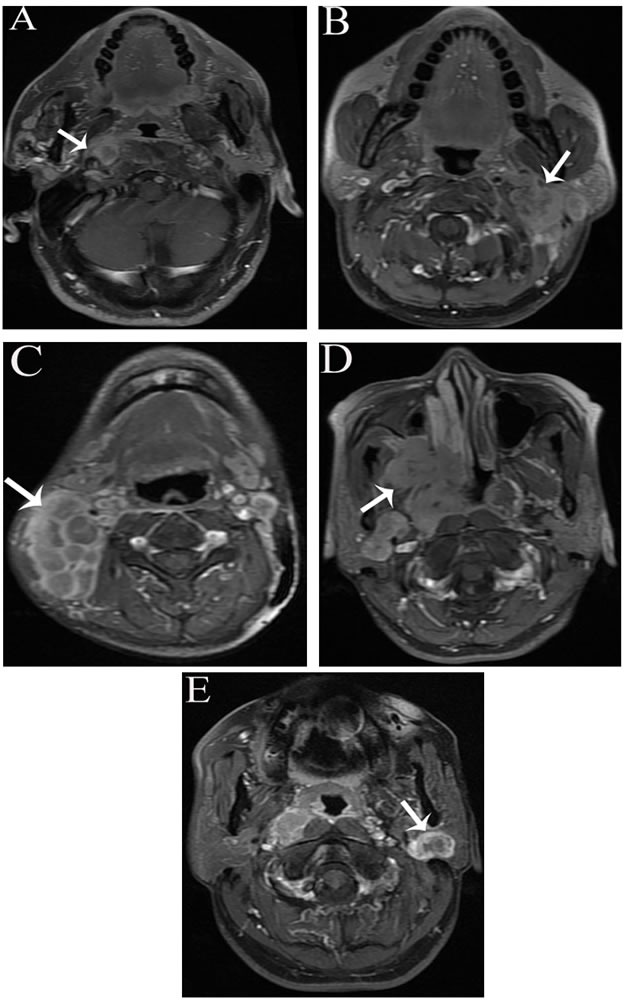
The characteristics of PLN spread on axial contrast-enhanced T1 fast spin-echo fat-suppressed (FSE fs) MRI A. Ipsilateral retropharyngeal lymph nodal extracapsular spread; B. ipsilateral level II nodal extracapsular spread; C. ipsilateral giant cervical nodes; D. ipsilateral extensive parapharyngeal space involvement; and E. solitary parotid lymphadenopathy.
